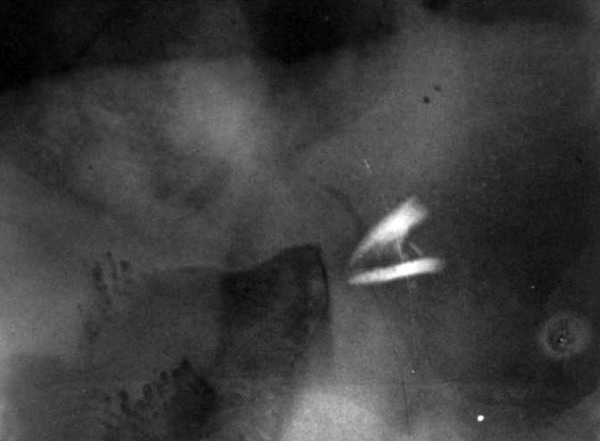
Radiographic image showing tooth fragment in the lower lip
